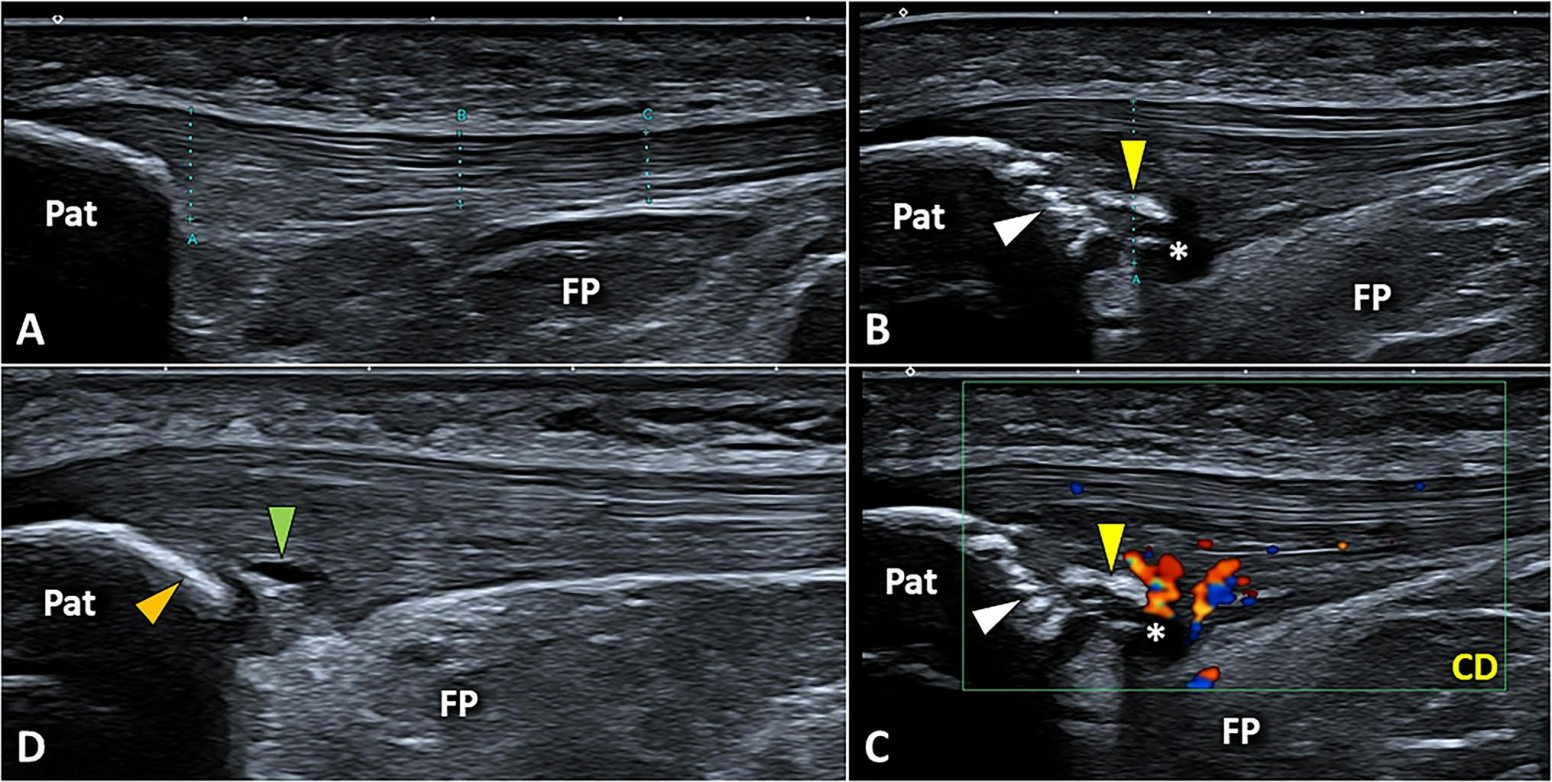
Normally (A), the patellar tendon presents a fibrillar echotexture in the longitudinal scan; instead, a hypoechoic thickening of the deep and proximal fibers *(white asterisk)*, lamellar calcifications *(yellow arrowhead)*, and cortical irregularities *(white arrowhead)* of the inferior pole of the patella *(Pat)* can be commonly observed in padel players with patellar tendinopathy (B). The color Doppler *(CD)* depicts neo-vessels (C) originating from the Hoffa fat pad *(FP)* and infiltrating the hypoechoic degenerated tendon tissue *(white asterisk)*. More rarely, an isolated longitudinal tear of the PT *(green arrowhead)* can be identified with a regular enthesis *(orange arrowhead)* (D)
